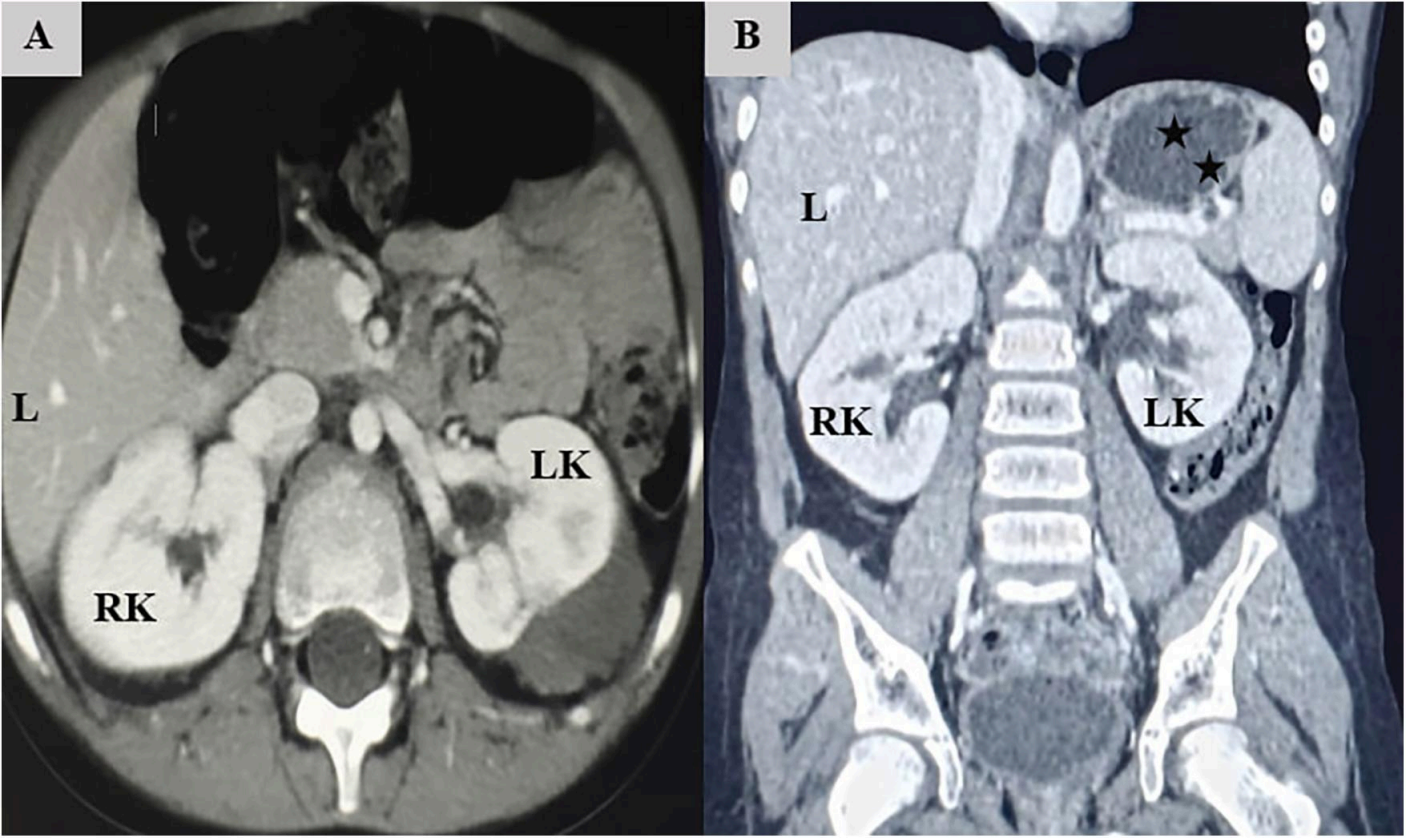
Follow-up computed tomography (CT) after 6 months of neoadjuvant chemotherapy. (A) Axial image showing significant reduction in tumor size and restoration of the fat plane between the right kidney (RK) and the liver (L). (B) Coronal image demonstrating the residual complex cystic mass in the upper pole of the left kidney (LK) (asterisks), and the well-preserved parenchyma of the right kidney (RK).
